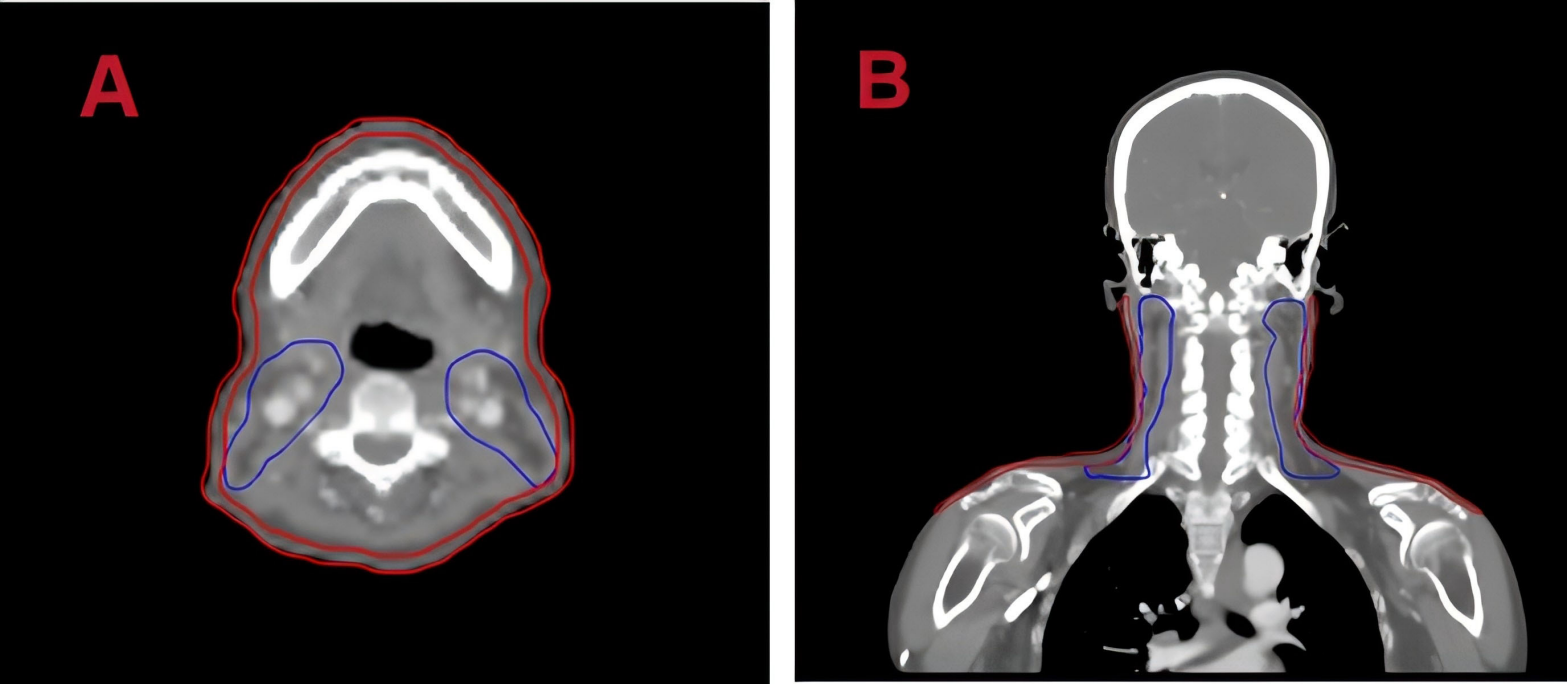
Regions of interest (ROI) on planning CT images. (A) Axial and (B) coronal views with skin delineated in red.
